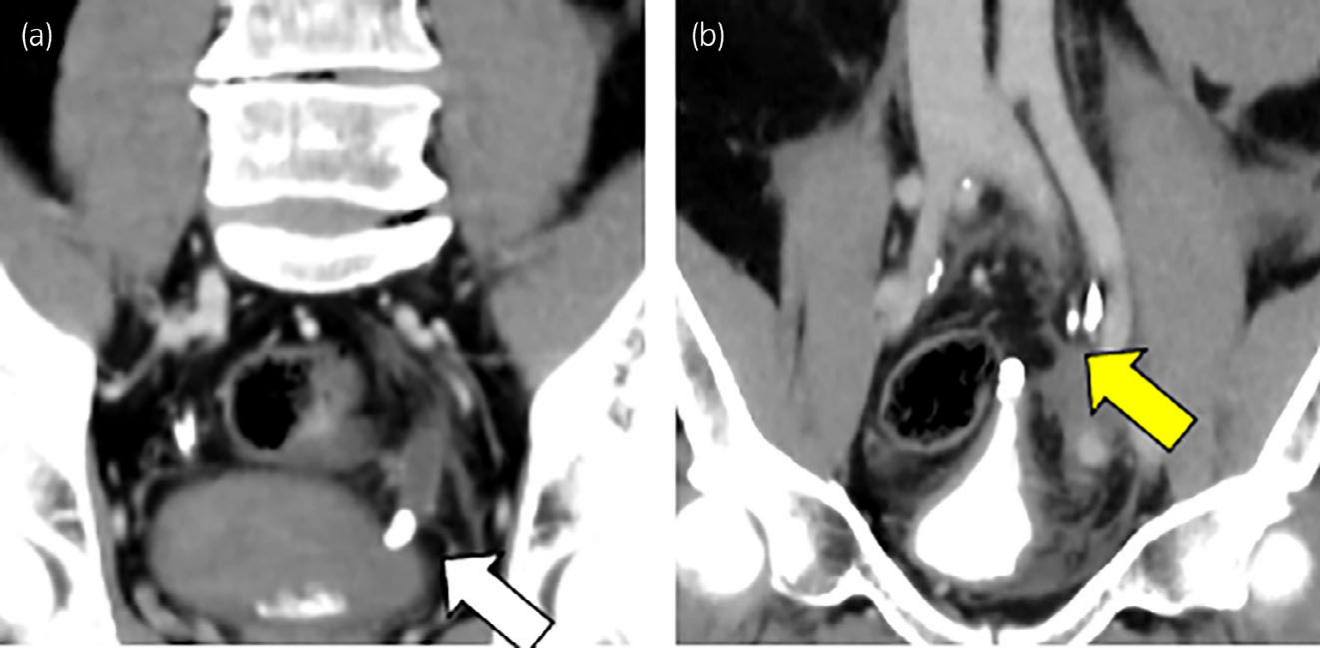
(a) Ureteric calculus of ~11 mm in the vesicoureteral transition area (white arrow). (b) Enhanced CT with excretory phase showing incompletely duplicated ureter (yellow arrow).
